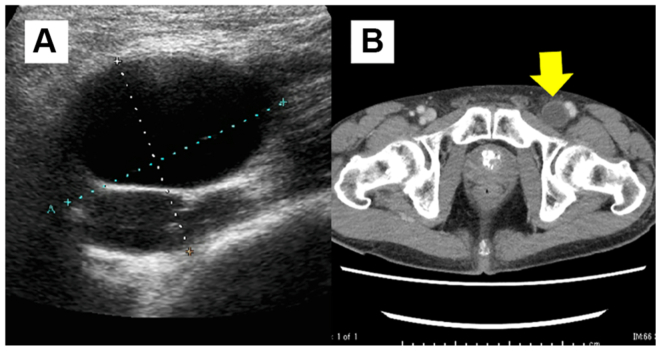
Preoperative imaging of the femoral vein adventitial cystic disease (ACD). (A) Duplex ultrasound image showing a multilocular cystic lesion adjacent to the femoral vein. (B) Contrast-enhanced computed tomography (CT) scan confirming a cystic mass compressing the vein (*arrow*).
